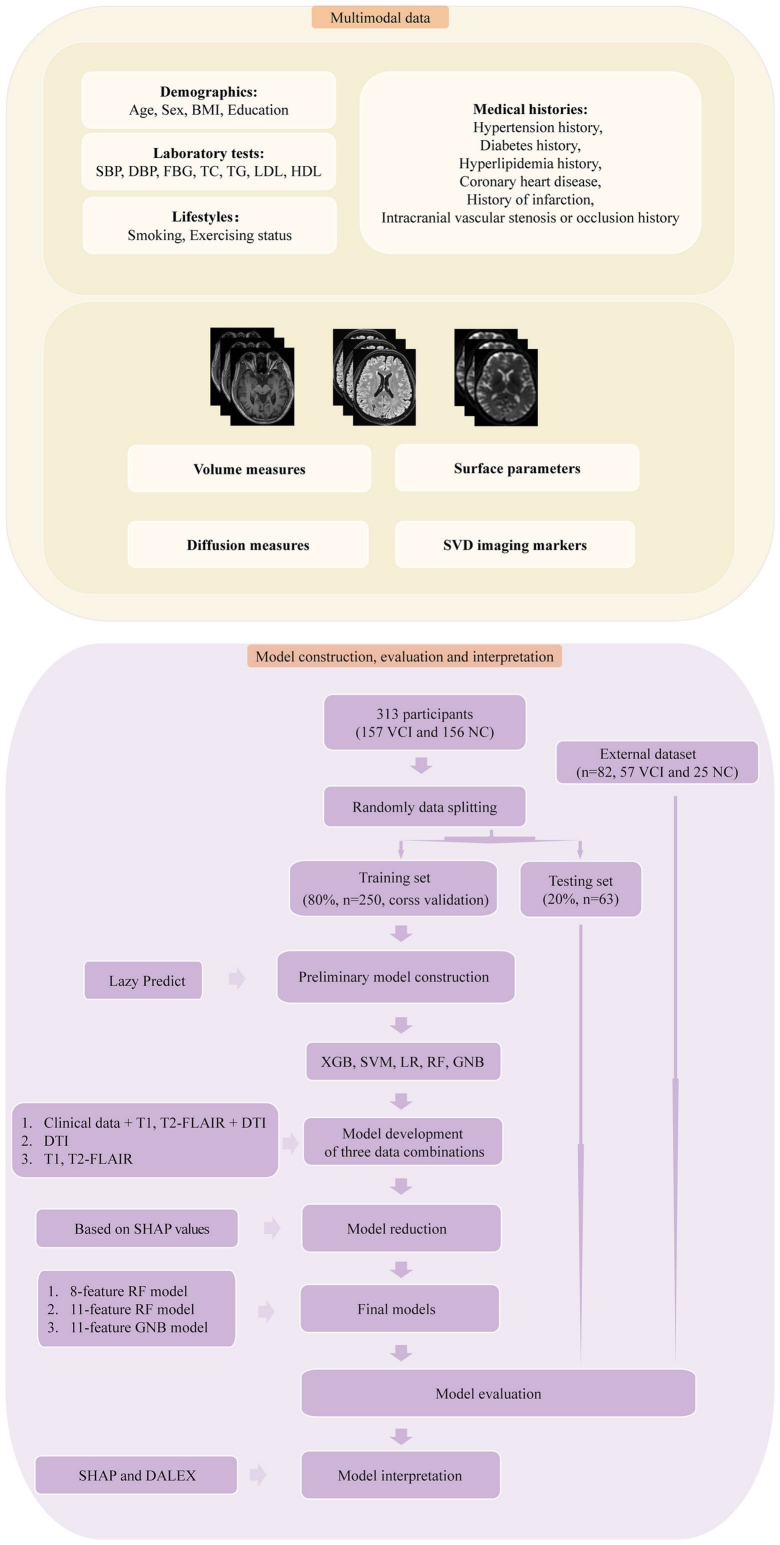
The framework for multimodal data processing, model construction, model evaluation and model interpretation. Multimodal data included various inputs, including clinical data and multimodal MRI. VBM, SBM and DTI measures were extracted from T1 and DTI, while SVD imaging markers were derived from T1 and T2-FLAIR. The models were developed using four ML algorithms and three combinations of data modalities. Model reduction was performed based on SHAP values. Subsequently, the final models underwent evaluation, external validation, and further interpretation.
